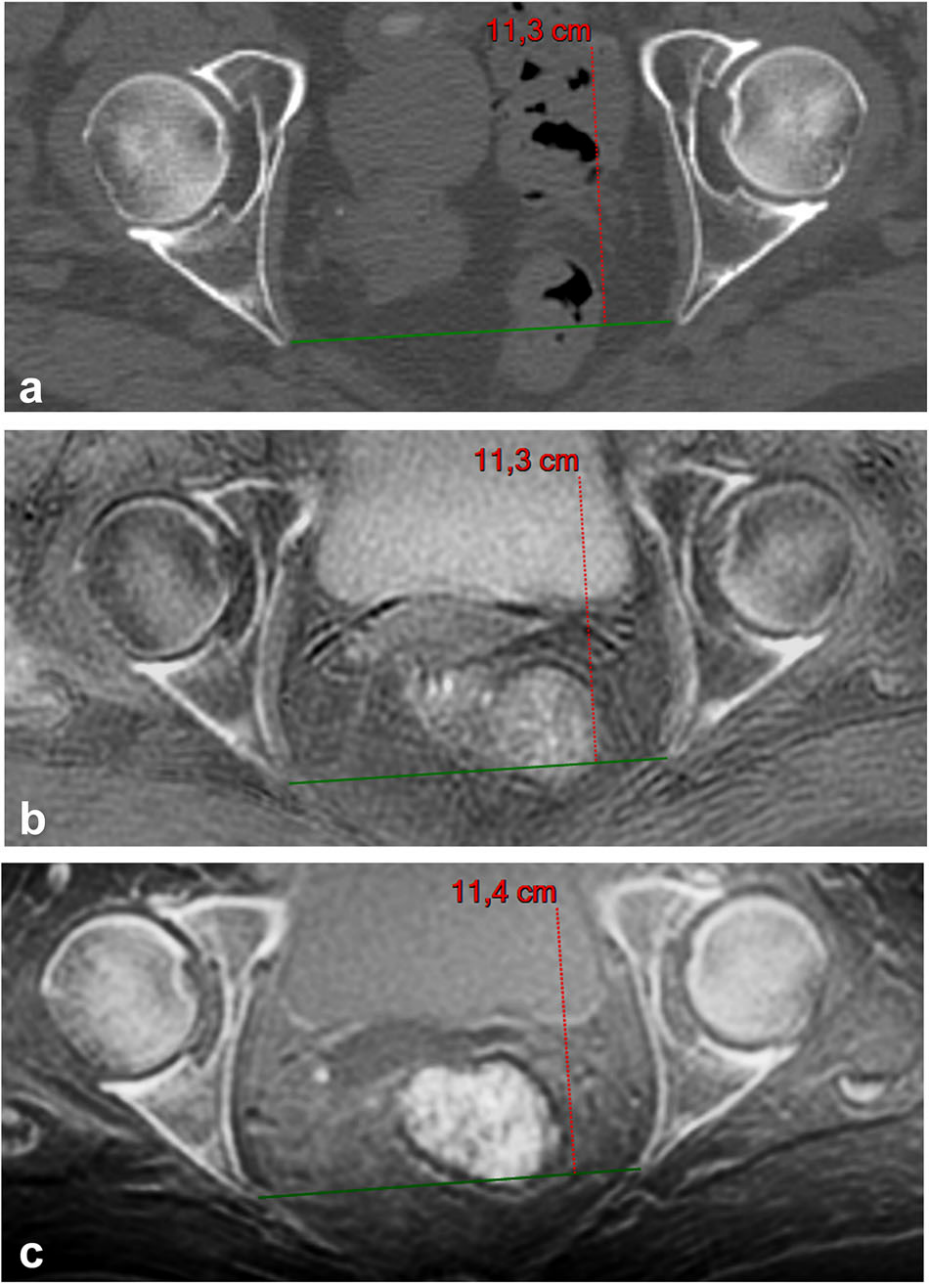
A 67-year-old woman with multiple myeloma, axial reformatted images for measuring the interspinous distance (green line): (a) reference computed tomography; (b) inverted gray-scale zero echo time MRI sequence; and (c) inverted gray-scale black bone MRI sequence. MRI, Magnetic resonance imaging
